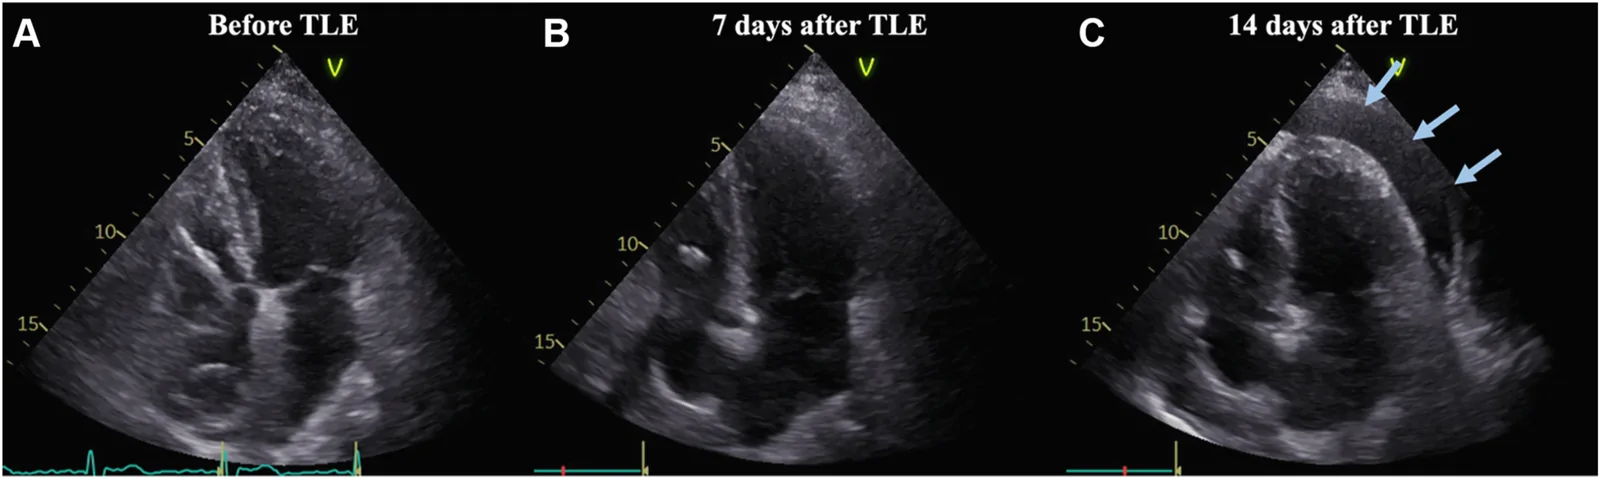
Serial transthoracic echocardiography images. A: On admission (preoperative). B: 7 days after TLE. C: 14 days after TLE. (*Blue arrows*) pericardial effusion. TLE = transvenous lead extraction.
